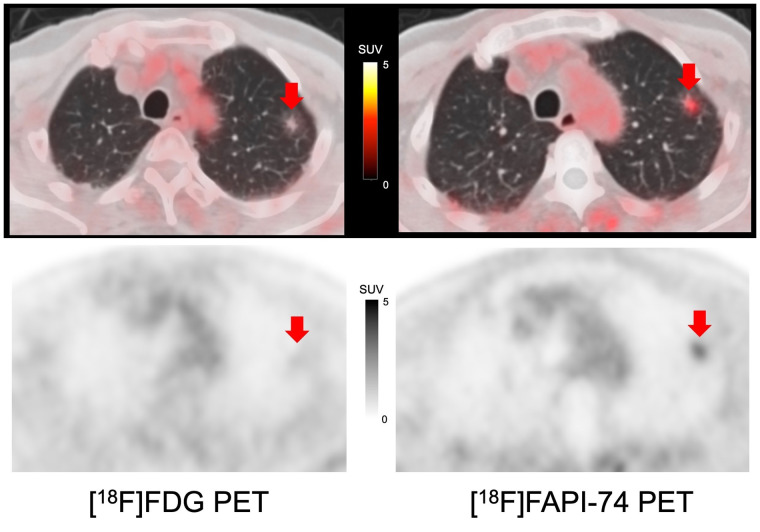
Comparison of PET/CT images on [^18^F]FAPI-74 PET and [^18^F]FDG PET of 80-y-old man with lung adenocarcinoma with ground-glass opacity (arrows indicate primary lesions). [^18^F]FAPI-74 PET showed clearer uptake than [^18^F]FDG PET (SUV_max_ of primary lesion is 2.5 and 1.2, respectively).
